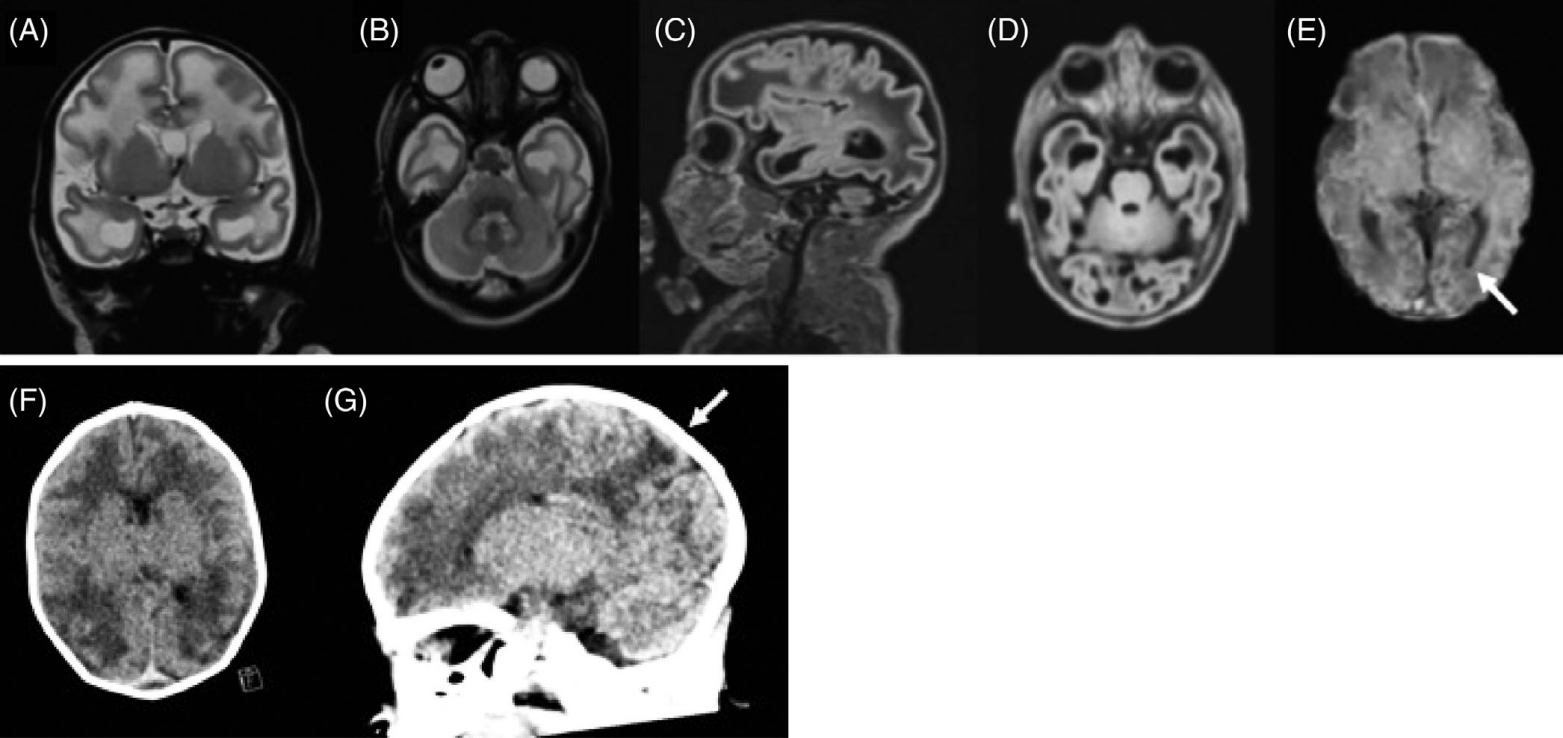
Cerebral imaging of PLPHP deficient patients. A‐E, Cerebral MRI of patient 1 at 3 days of age: Coronal T2W (A), axial T2W (B), sagittal flair (C), and axial flair (D) indicated a global delay of brain development with dysmature cerebral hemispheres with broad gyri and shallow sulci. Cortical folding was delayed by ~ 2 to 6 weeks. Myelination of the white matter was delayed including delayed myelination of central white matter structures (posterior limb of the internal capsule). The corpus callosum was abnormally thin. There were subcortical and deep white matter edema. Cerebellum appeared unremarkable. SWI (susceptibility weighted imaging; E) showed a small hemosiderin deposit in the left occipital horn (arrow). F and G, Brain CT of patient 2 at 4 days of age showed decreased attenuation in the white matter, accentuated in subcortical areas of especially the parietal lobes (arrows) with broad gyri, compatible with edema. The ventricular system was symmetric and of normal size with a cavum Vergae. Neither periventricular cysts nor hemorrhages were seen
